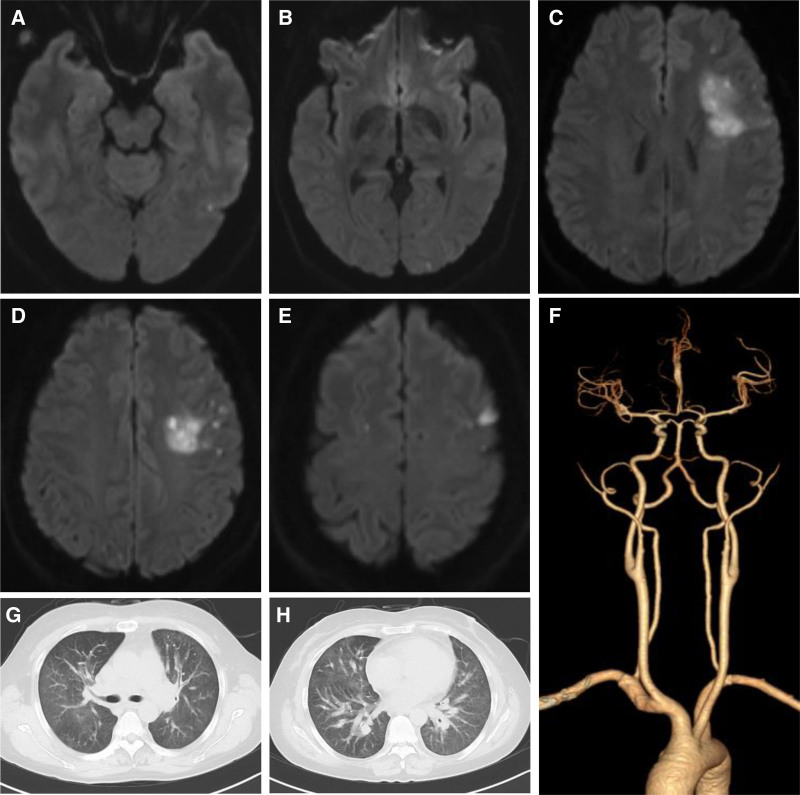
Images of Case 2. (A) MRI shows acute cerebral infarctions in the left temporal lobe. (B) MRI shows acute cerebral infarctions in the left occipital lobe. (C) MRI shows acute cerebral infarctions in the left frontoparietal lobe and bilateral occipital lobes. (D) MRI shows acute cerebral infarctions in the left frontoparietal lobe. (E) MRI shows acute cerebral infarctions in the bilateral parietal lobes. (F) Head and neck CTA showed no obvious abnormalities. (G) Chest computed tomography (CT): pulmonary parenchyma, tracheal wall edema and thickening, and mediastinal edema. (H) Chest CT: pulmonary parenchyma, tracheal wall edema and thickening, and mediastinal edema. CTA = computed tomography angiography, MRI = magnetic resonance imaging.
